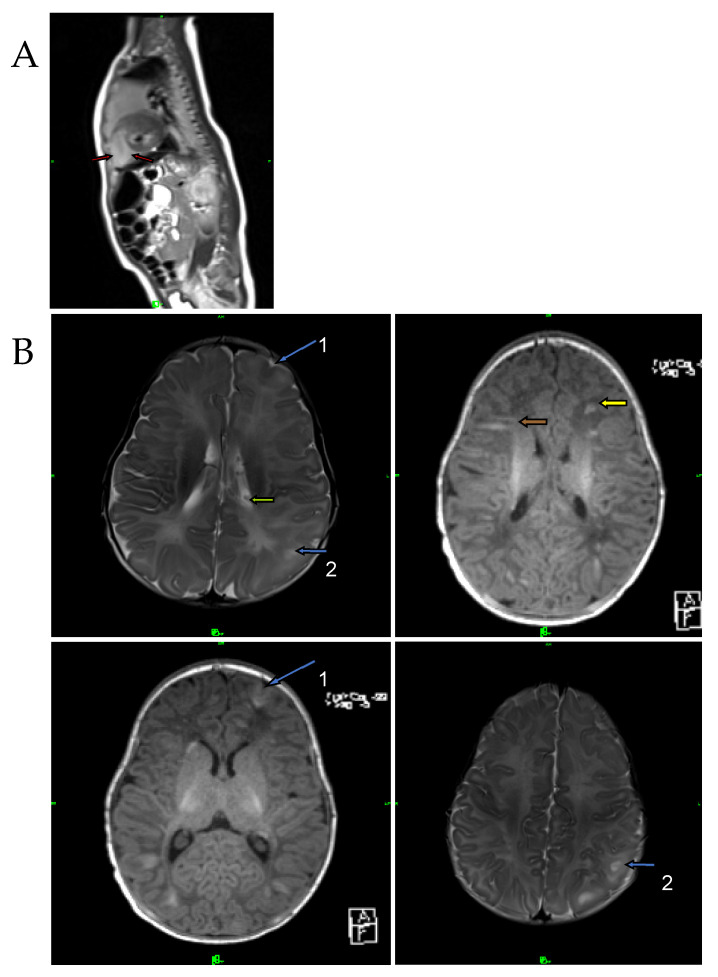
T1-weighted axial MRI image of the brain and T2-weighted sagittal MRI image of the heart at 2 months of age. (A) Red arrows represent cardiac rhabdomyoma. (B) Blue arrows show cortical tubers in the sulcal island (1) and in the gyral core (2); green arrow represents a subependymal nodule. Brown and yellow arrows show white-matter radial migration lines: straight band (brown), nonspecific conglomerate foci (yellow).
